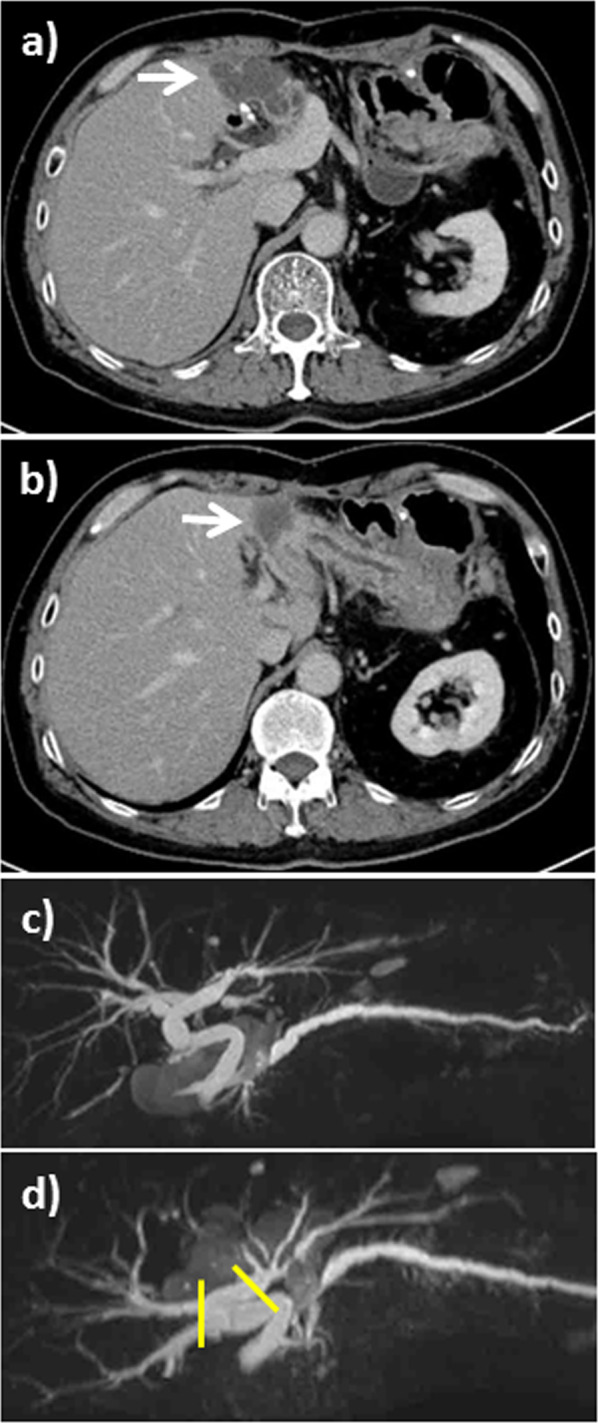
Preoperative enhanced computed tomography and magnetic resonance imaging. a The white arrow shows the recurrent tumor in the hepatic hilum. b The white arrow shows the recurrent tumor in the hepatic hilum. The main pancreatic duct was dilated. c Tumor invasion to the main pancreatic duct and dilatation of the main pancreatic duct on its caudal side. d The yellow lines show the resection line of bile ducts
